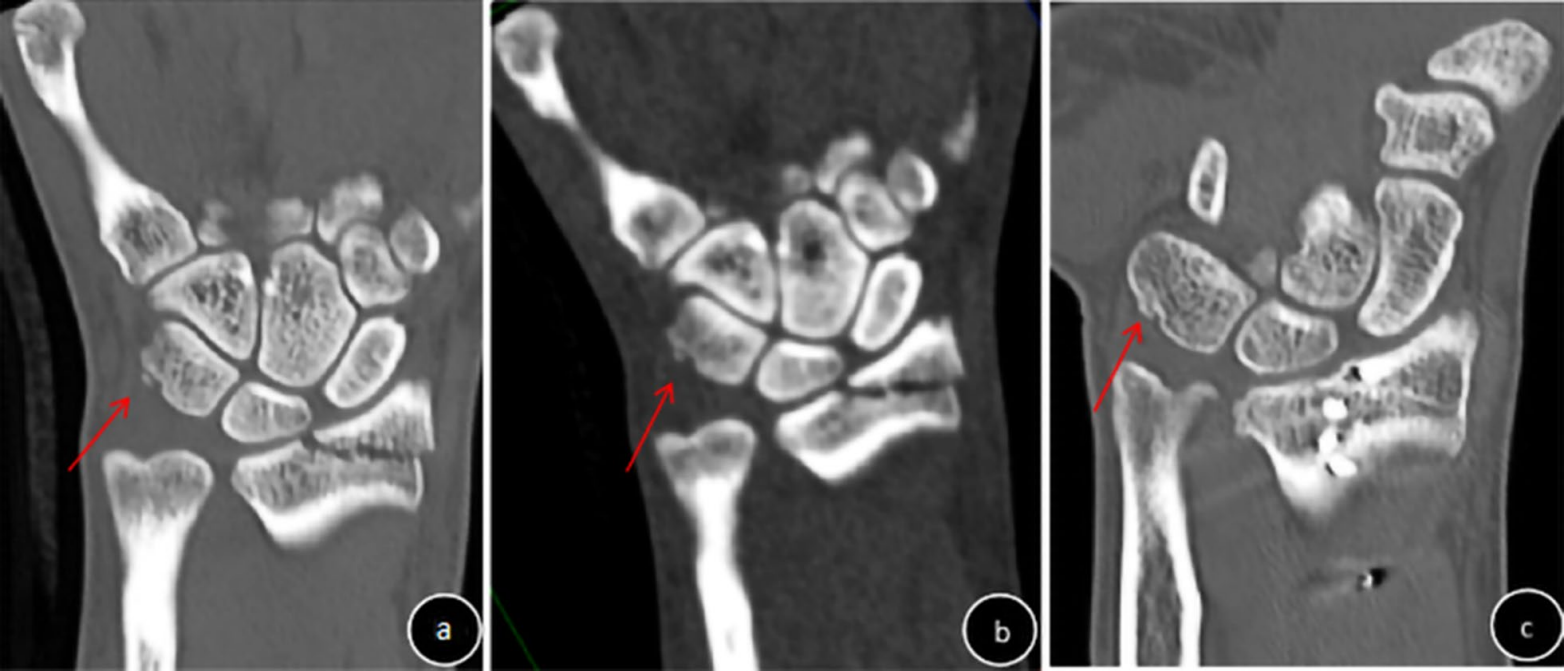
Triangular avulsion fracture of the wrist (red arrow). a SD-CT image; b ULD-CT image; c 3-month follow-up. SD-CT scan diagnosed an old fracture. ULD-CT scan diagnosed an avulsion fracture
